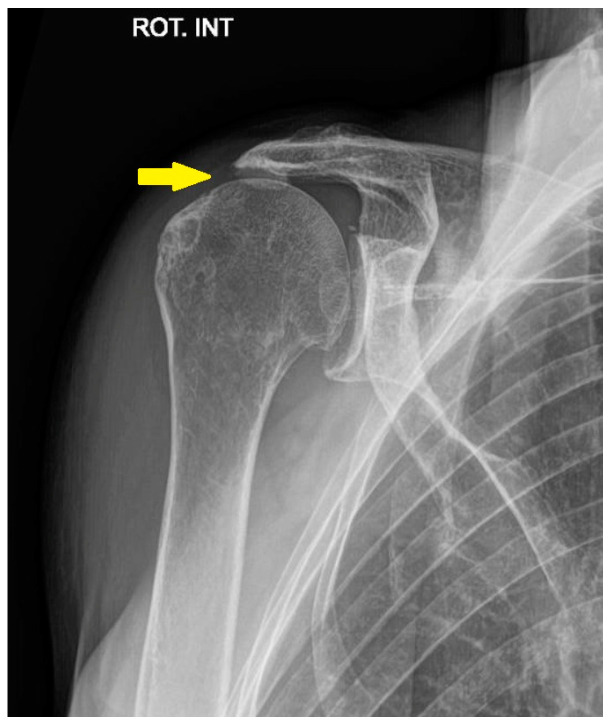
Rotator cuff arthropathy on the anteroposterior radiograph. The yellow arrow shows narrowing of the acromiohumeral space due to a posterosuperior rotator cuff tear.
